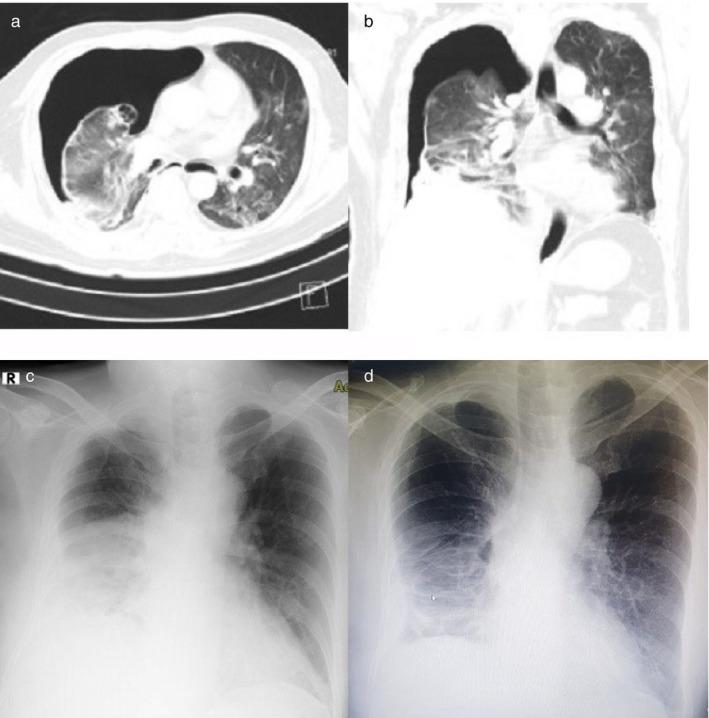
(a, b) Coronal and axial slices of thorax CT scan on April 22 showed ground‐glass opacity with a multifocal fibrotic band with improvement on left lung segment; Pneumothorax on superolateral right lung with right lung collapse; within right lung collapse, was seen ground‐glass opacity, consolidation, thick‐walled cavity with fibrosis, and emphysematous bullae with partially septated with medial and inferior segments of the right lung; minimal pleural effusion on the posterobasal of the right lung; paraseptal emphysema on 1st segment of the left lung. (c) The latest chest X‐ray before hospital discharge showed improvement of right lung pneumothorax and massive right lung fibrosis, and right pleura thickening, (d) chest X‐ray 2 weeks after hospital discharge showed residual but improved massive right lung fibrosis and right pleura thickening
